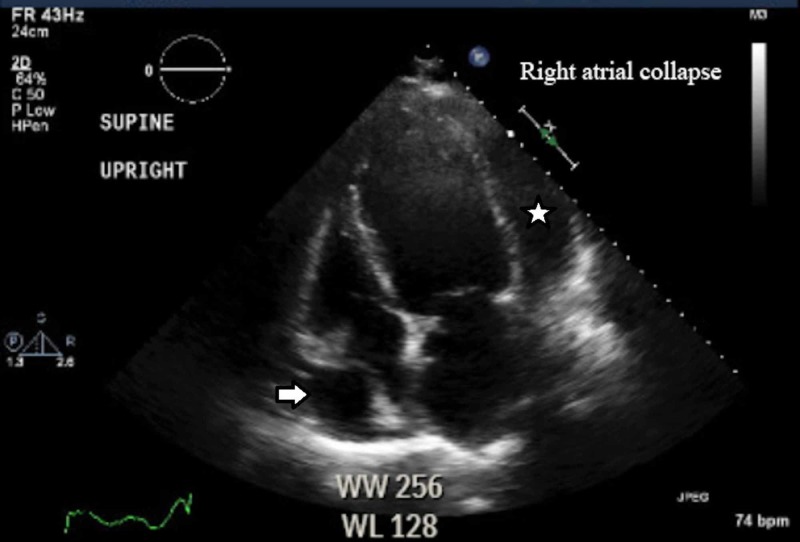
Echocardiogram. Image shows pericardial effusion (white star) and right atrial collapse (white arrow).
